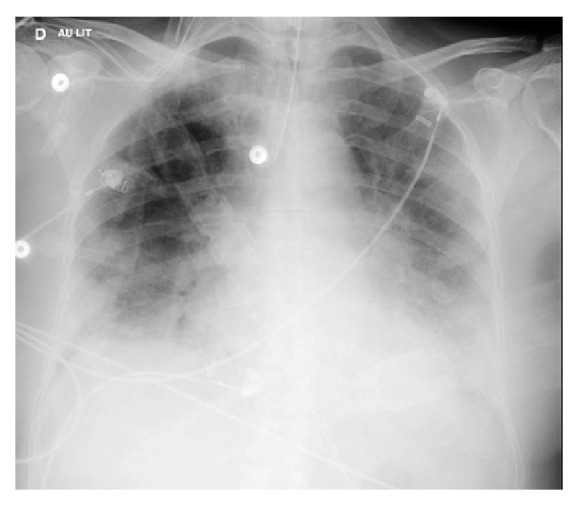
The chest X-ray (in supine position) on admission showing bilateral alveolar-interstitial syndrome with bilateral lower lobe consolidations.
